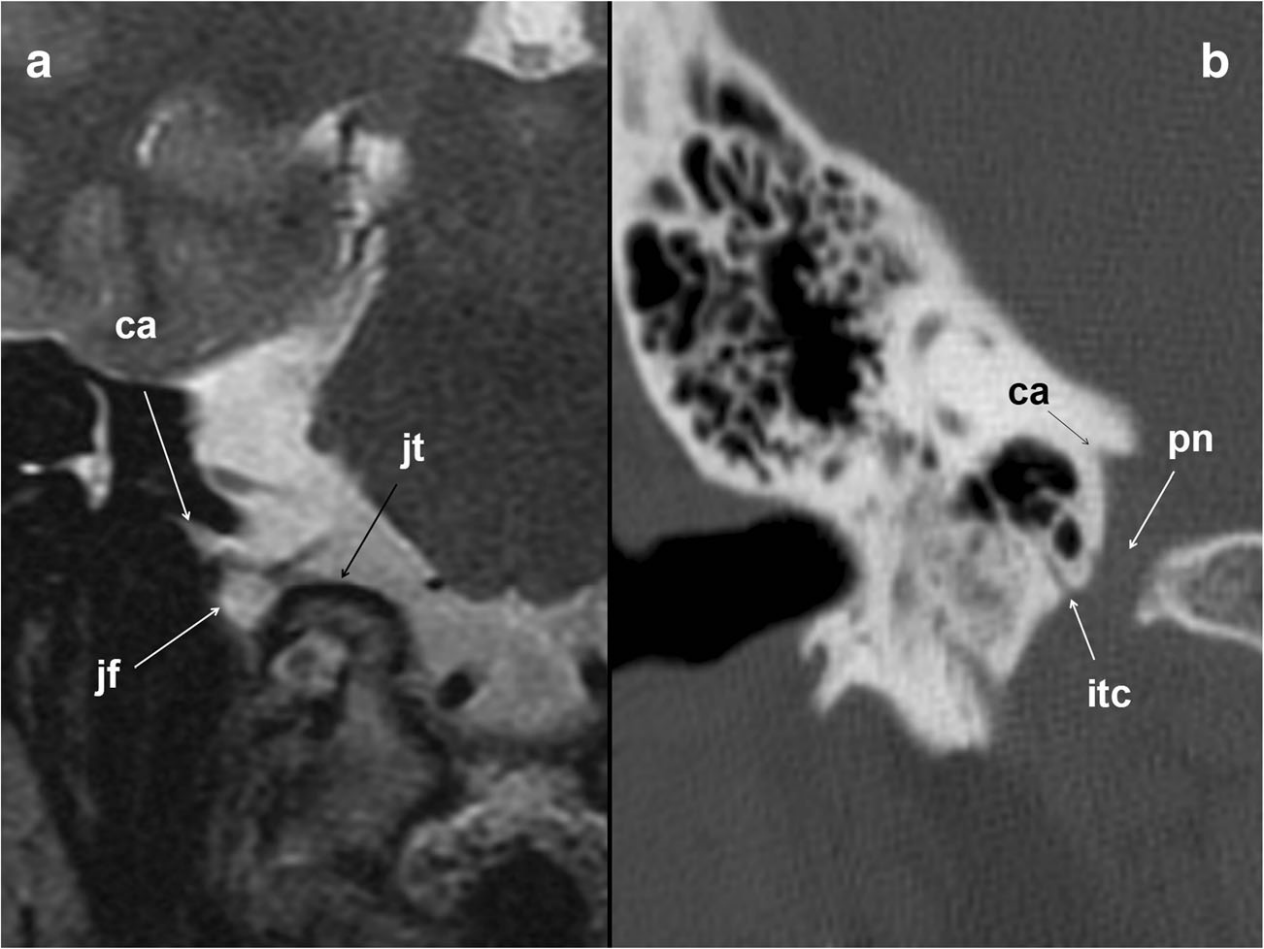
The glossopharyngeal nerve at the jugular foramen. a Coronal T2-weighted brain MRI at the level of the jugular foramen (jf). The image shows the relationship between the cochlear aqueduct (ca) above, and the jugular foramen and jugular tubercle (jt) below. b Skull base CT scan; coronal reconstruction of the right temporal bone, bone window. The image shows the inferior tympanic canaliculus (itc) for the inferior tympanic or Jacobson’s nerve at the jugular spine. ca: cochlear aqueduct; pn: pars nervosa
